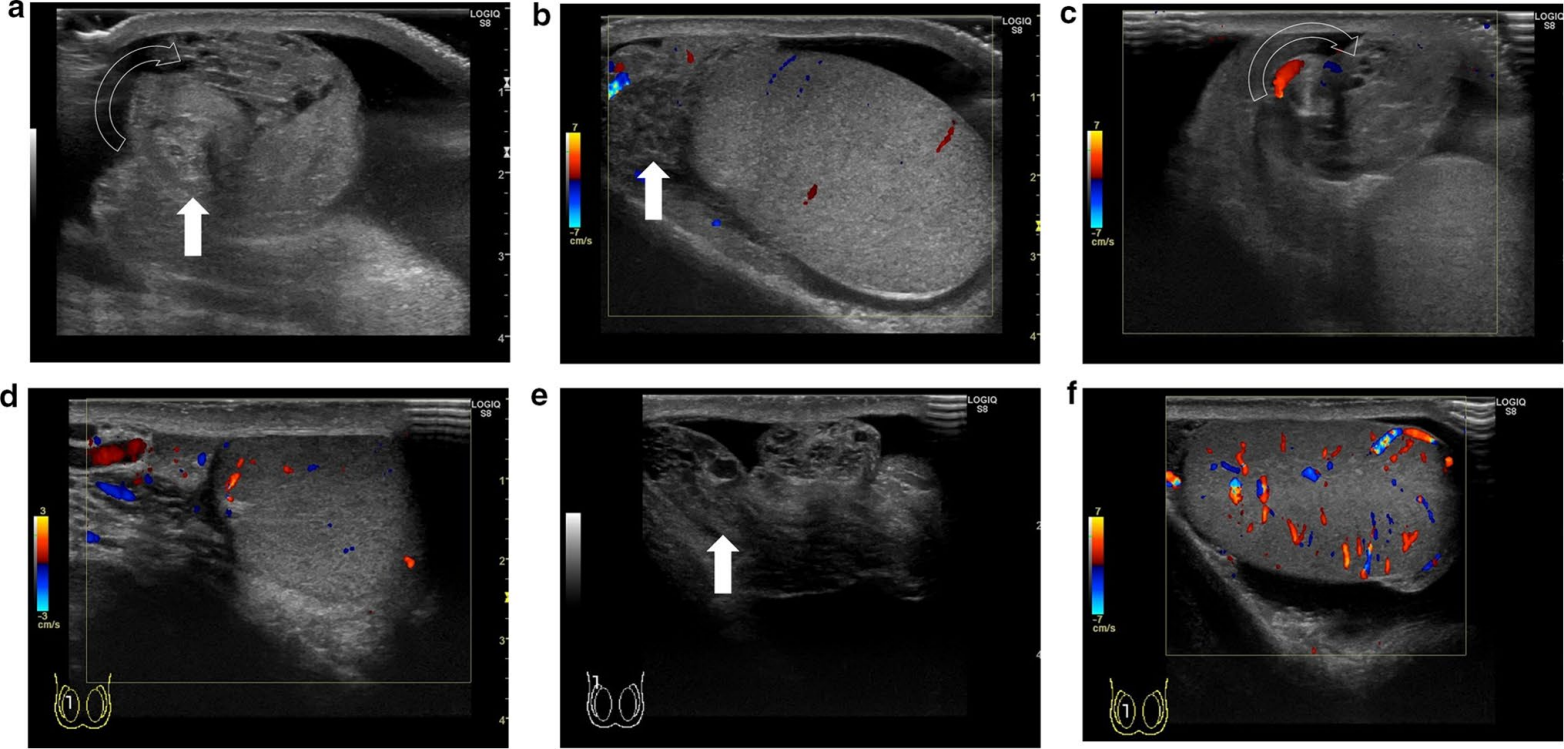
Ultrasonographic images of a 14-year-old boy with right testicular torsion. a The whirlpool sign is evident. b Swelling of the right testis and decreased vascular flow are evident. c The whirlpool sing is preserved after manual detorsion. d The vascularity within the affected testis is greater than that before manual detorsion, but it is not hypervascular. e The whirlpool sign is diminished after subsequent manual detorsion. f Hypervascularity within the testis is evident
